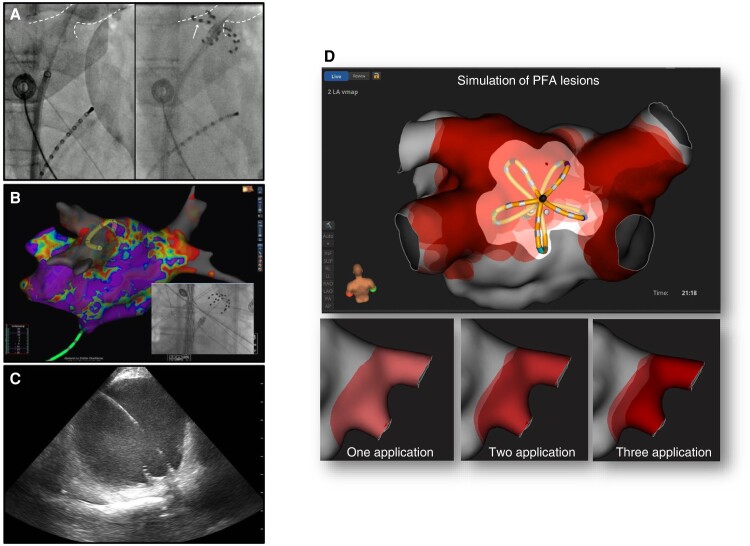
Pulsed field ablation practical considerations. Pulsed field as an energy form is less contact force sensitive, since the electrical field spreads into tissues surrounding the catheter. However, pre-clinical data show that tissue contact has a direct effect on lesion depth and durability. Currently, catheter visualization, navigation, and contact assessment are primarily based on fluoroscopy, intracardiac echocardiography, or simplified impendence-based 3D mapping. In future, sophisticated concepts regarding catheter navigation and contact assessment will evolve (*Figure 4*). If deep sedation instead of general anaesthesia was used, PFA typically stimulates skeletal muscles and the phrenic nerve. In this scenario, a stabilizing guidewire may be beneficial reducing the risk of device dislocation but needs to balanced vs. the risk of mechanical trauma. (*A*) The pentaspline device position can be assessed in comparison with selective PV angiograms. In addition, the interaction between splines and tissue adds valuable information regarding local contact (arrow). (*B*) Alternatively, the pentaspline devices can be visualized within a 3D mapping system with impedance-based catheter localization. However, the device is displayed only as a circular structure, reducing catheter positioning accuracy. (*C*) Real-time catheter localization may be also assessed using intracardiac echo: besides embedded costs, this seems to be a viable alternative to reduce X-ray exposure (courtesy of Bart Moulder). (*D*) Future 3D mapping integration and lesion visualization of repetitive pentaspline applications (courtesy of Melanie Gunawardene). PFA, pulsed field ablation.
